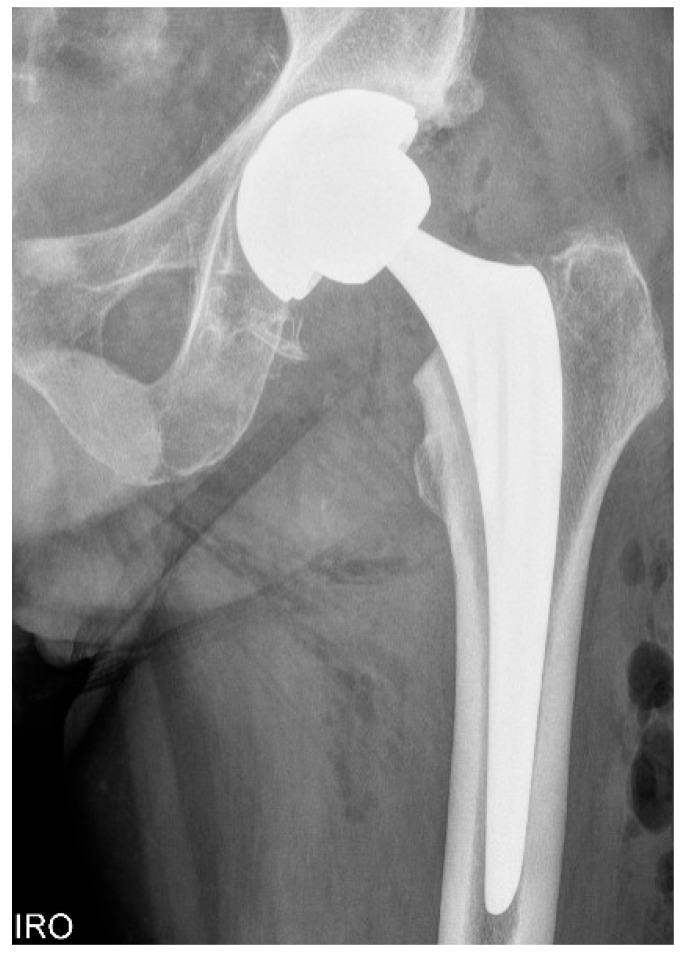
AP X-ray of a pelvic overview 12 weeks after implantation of a cementless hip prosthesis after femoral head necrosis.
